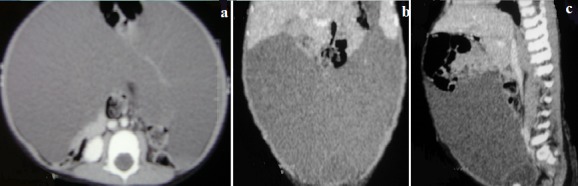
Computed tomography images shows a huge abdominal cystic mass measuring 35cm x 25cm x 15, with a septum that occupied nearly the entire abdominal cavity: A) transversal section; B) coronal section; C) sagittal section
